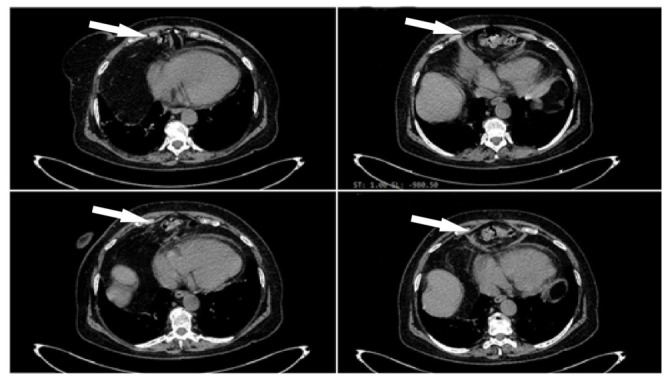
Chest CT imaging: right anterior diaphragmatic hernia, containing adipose tissues and the hepatic flexure of the colon.
